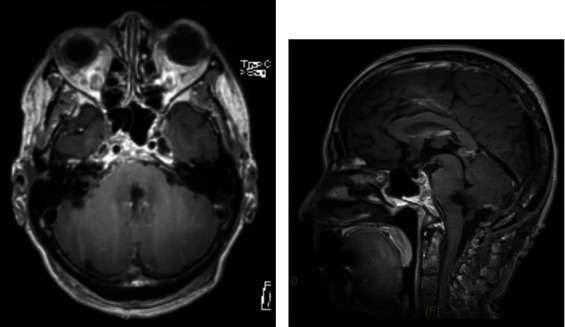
(a) Postoperative contrast-enhanced MRI confirms that the metastasis was totally removed. (b) The remaining medulla and pons tissues have filled the space, except for a very thin gap.
